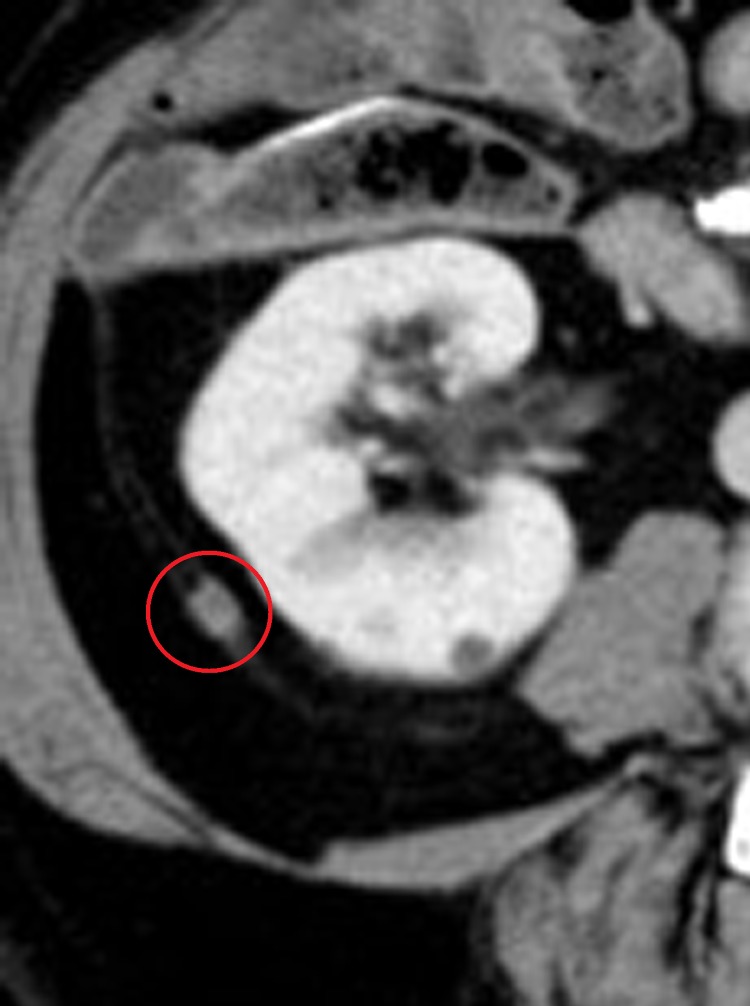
CT showed a solitary tumor in the right pararenal region of the retroperitoneal space 5 years after the operation (*red circle*).
